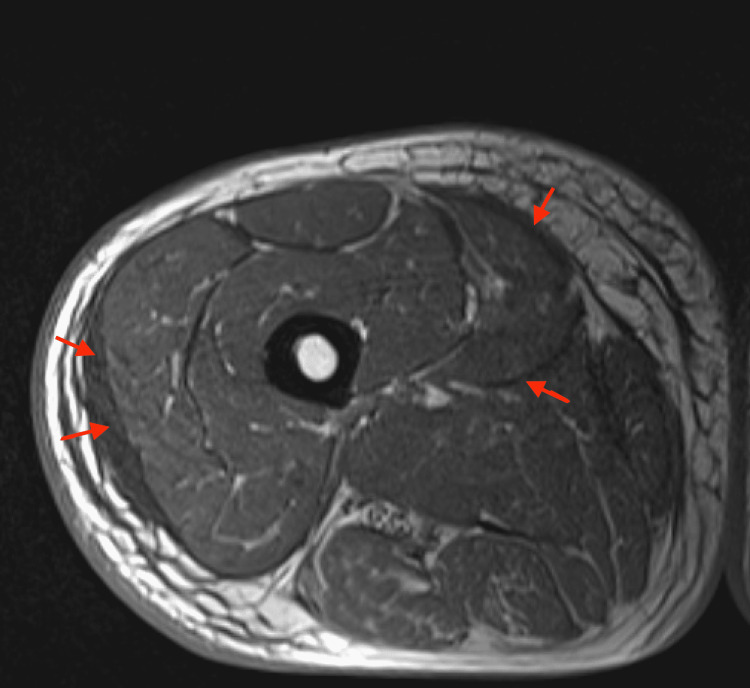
MRI of thigh T1 sequence, red arrows demonstrating diffuse subcutaneous and myofascial edema without focal abscess or necrosis.
